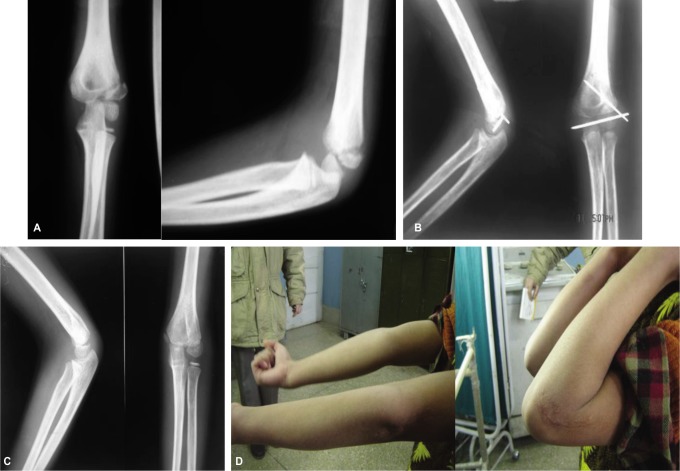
Showing the lateral condyle fracture in an 8 year old boy [A]. Open reduction and fixation was done after 6 weeks [B]. Final radiograph and clinical range of motion at 1 year [C and D].
